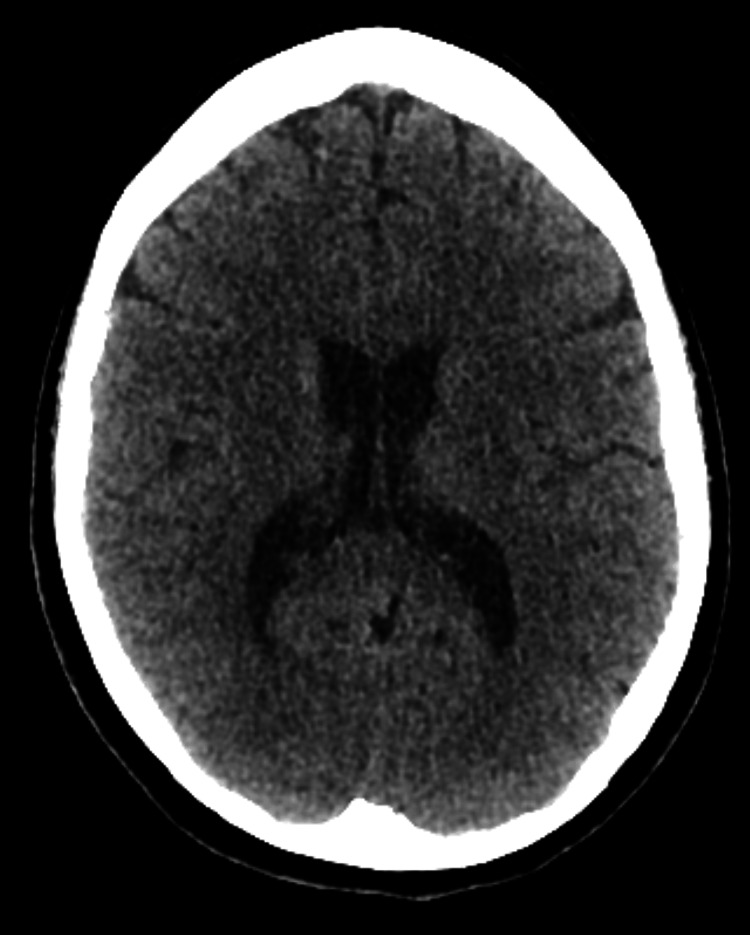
CT of the head: normal with no signs of stroke or intra-cerebral lesion.
